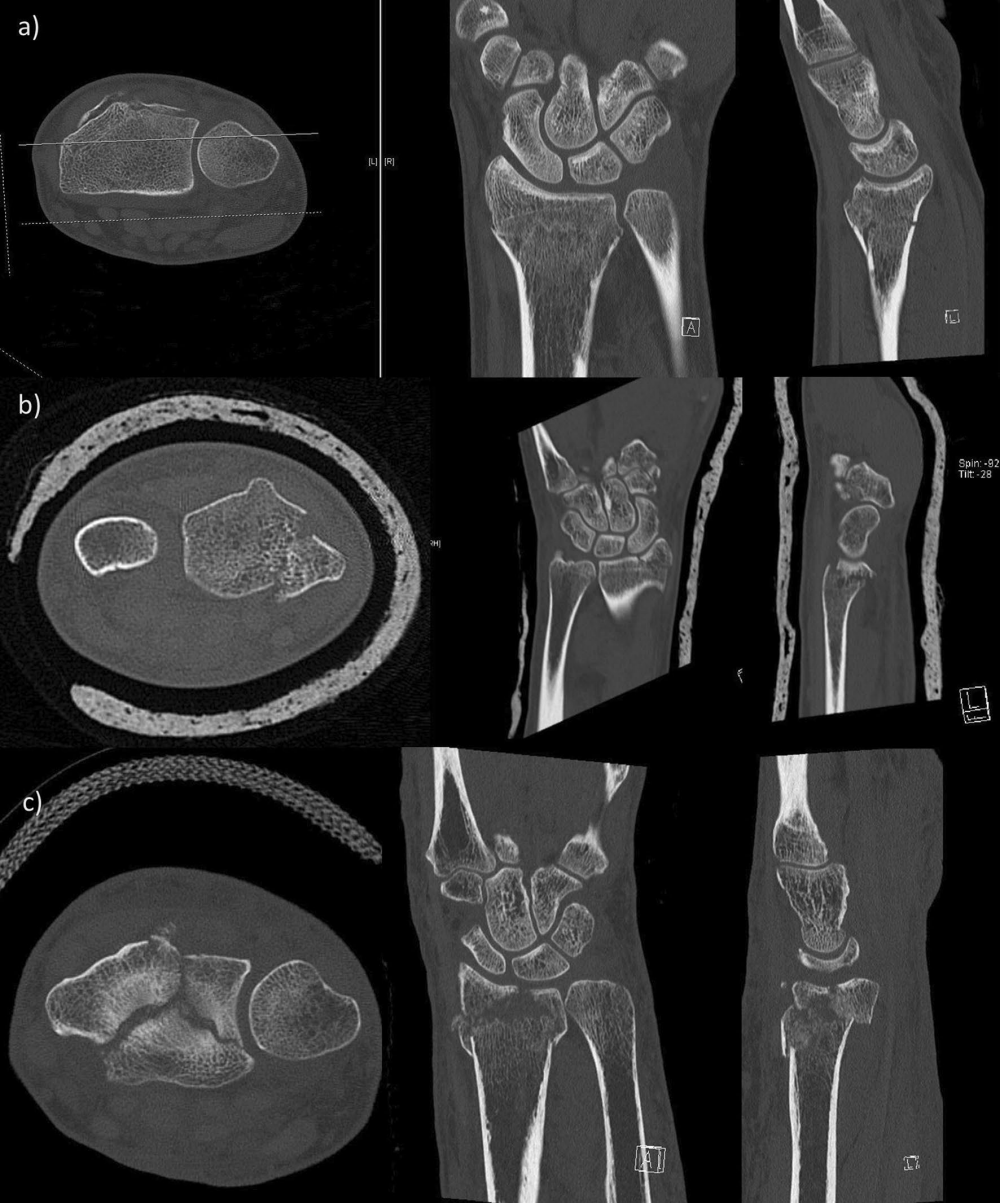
Example of CT scans used in the studies. Students were shown several slices and planes printed. a Distal radius fracture type a, b fracture AO b, and c fracture type c
